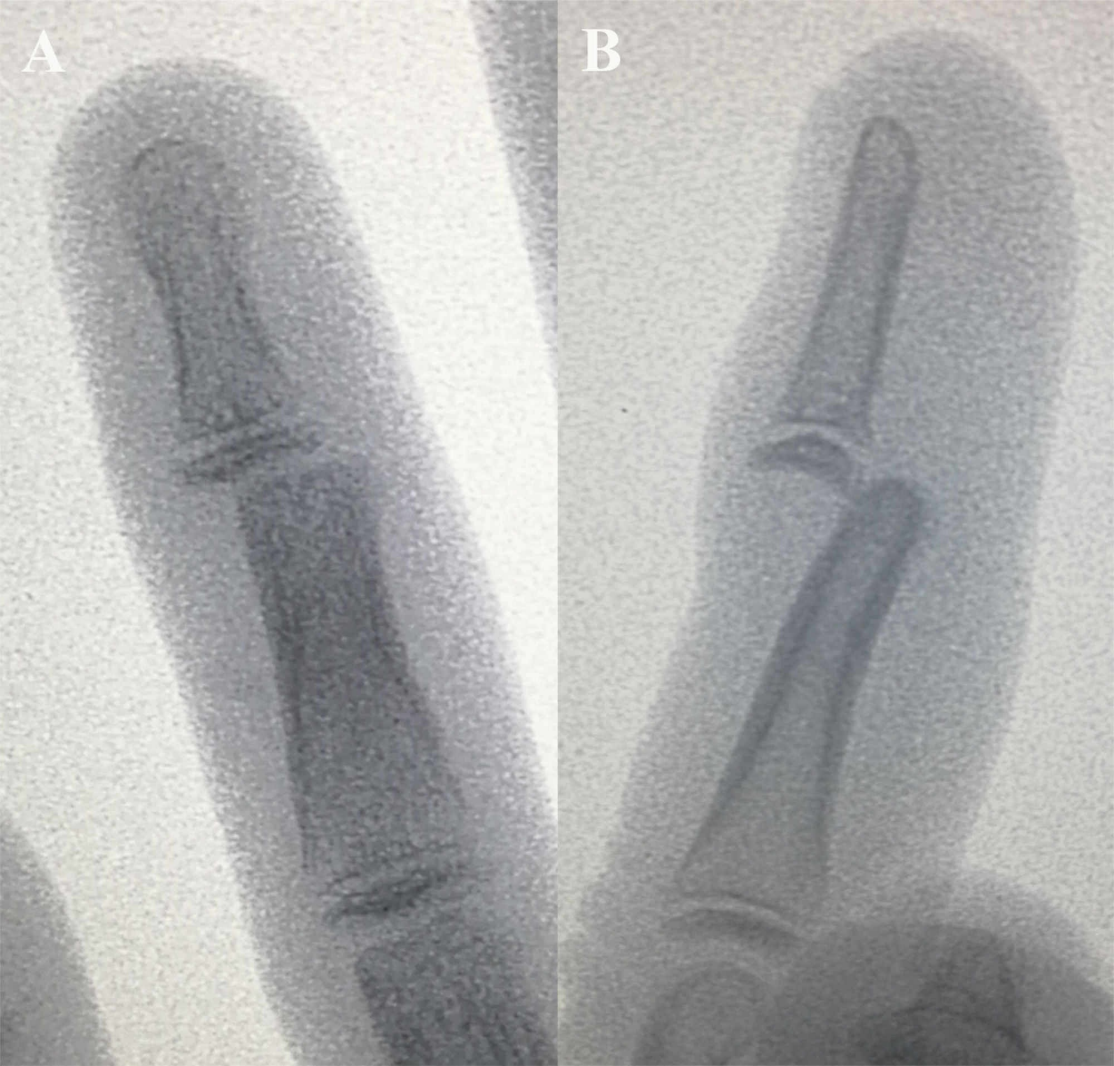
Preoperative radiographs revealed straight dorsal dislocation of the distal phalanx of the left ring finger in posteroanterior (A) and lateral (B) views
